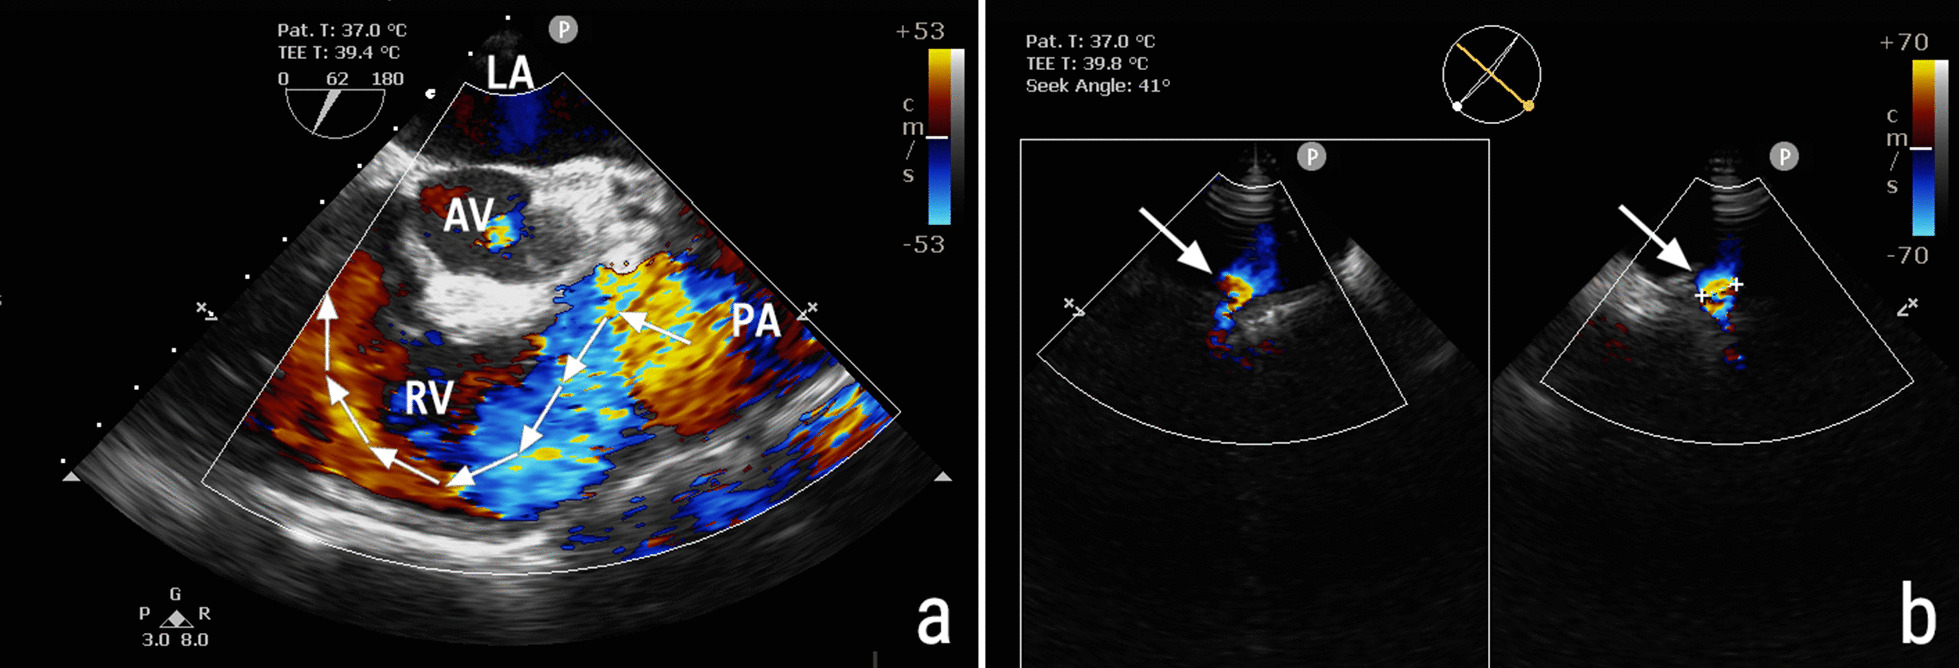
Transesophageal echocardiography. a Color Doppler imaging shows severe pulmonic valve regurgitation with an eccentric jet. The jet’s path is marked with seven arrows. b Color Doppler biplane imaging shows patent ductus arteriosus (*arrow*). *LA* left atrium, *AV* aortic valve, *RV* right ventricle, *PA* pulmonary artery
